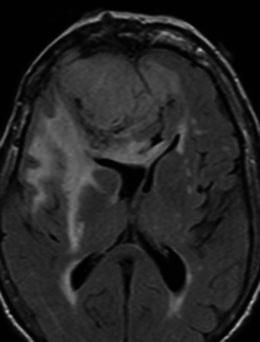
Axial FLAIR sequence in an 81-year-old woman with confusion and falls demonstrates a large extra-axial mass that is isointense to grey matter and is predominantly located within the right frontal lobe but crosses the midline falx anteriorly to indent the left frontal lobe. It induces substantial vasogenic oedema within the right frontal lobe, around the right basal ganglia and through the genu of the corpus callosum to the left forceps minor
